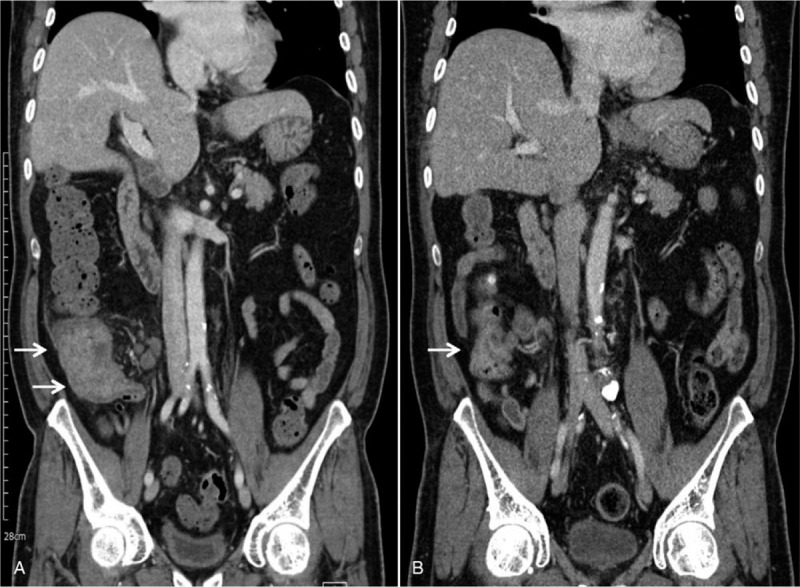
Abdominal computed tomography (CT) findings. (A) Initial abdominal CT scan shows a mass which is located just distal to ileocecal valve (arrows), with the formation of adjacent multiple lymphadenopathy. (B) Abdominal CT obtained at 9 months after antituberculous chemotherapy shows marked resolution of colonic tuberculosis (arrow). CT = computed tomography.
